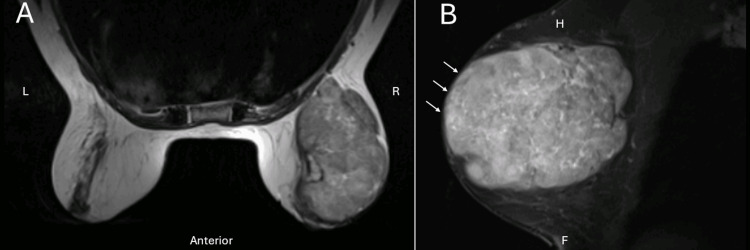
Magnetic resonance imaging of the breast (T2-weighted scan) in axial (A) and sagittal (B) plane showing a lesion occupying almost the entire right breast, with well-defined lobulated contours, measuring 93x71x82 mm and contact with the pectoral muscle and the skin surface (white arrows). F: foot direction; H: head direction; L: left direction; R: right direction
